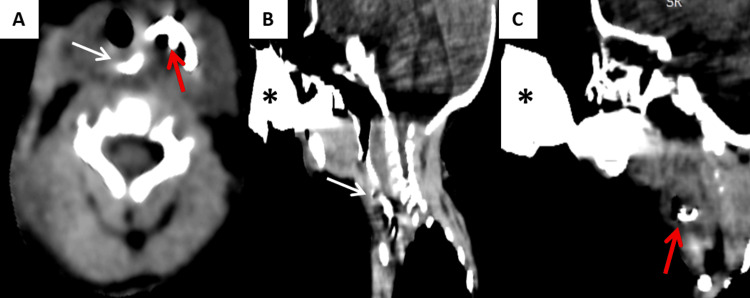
Cervical CT scan with oral contrast administered Cervical CT scan with oral contrast administered via feeding bottle (*), axial (A) and sagittal reconstructions (B–C) demonstrates opacification of the cervical collection (red arrow) during the passage of contrast along the esophagus (white arrow), confirming a communication between the esophageal lumen and the cervical cystic mass.
